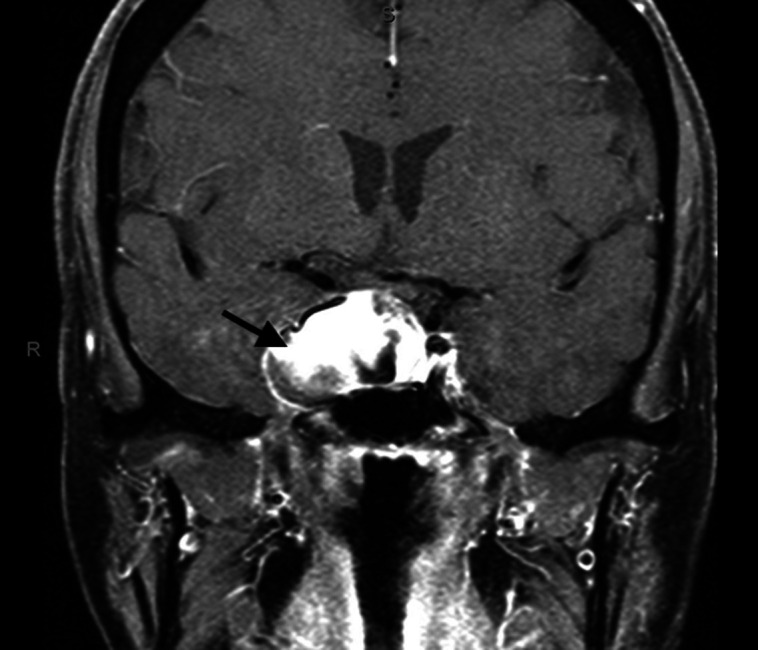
T1-weighted magnetic resonance of the brain revealed a large, heterogeneous right sellar mass (black arrow) involving the right cavernous sinus.
